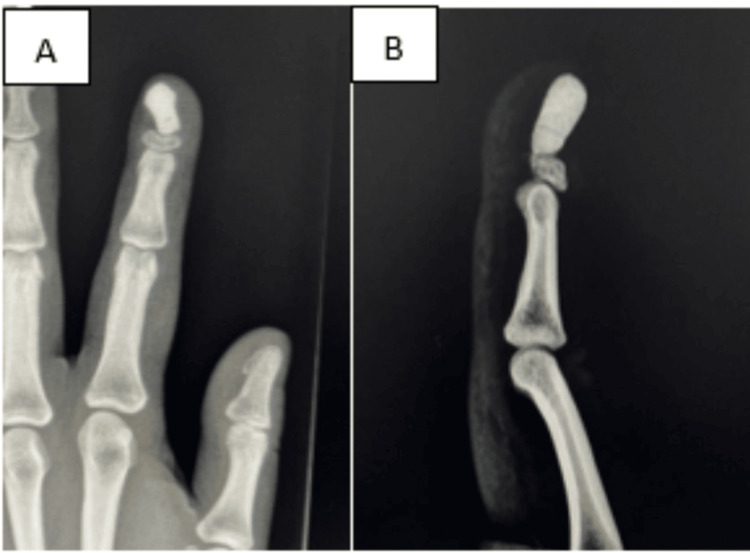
(A and B): Plain radiograph of left index finger showing malalignment of the bone cement over the distal phalanx impinging over the dorsal surface of the fingertip.
